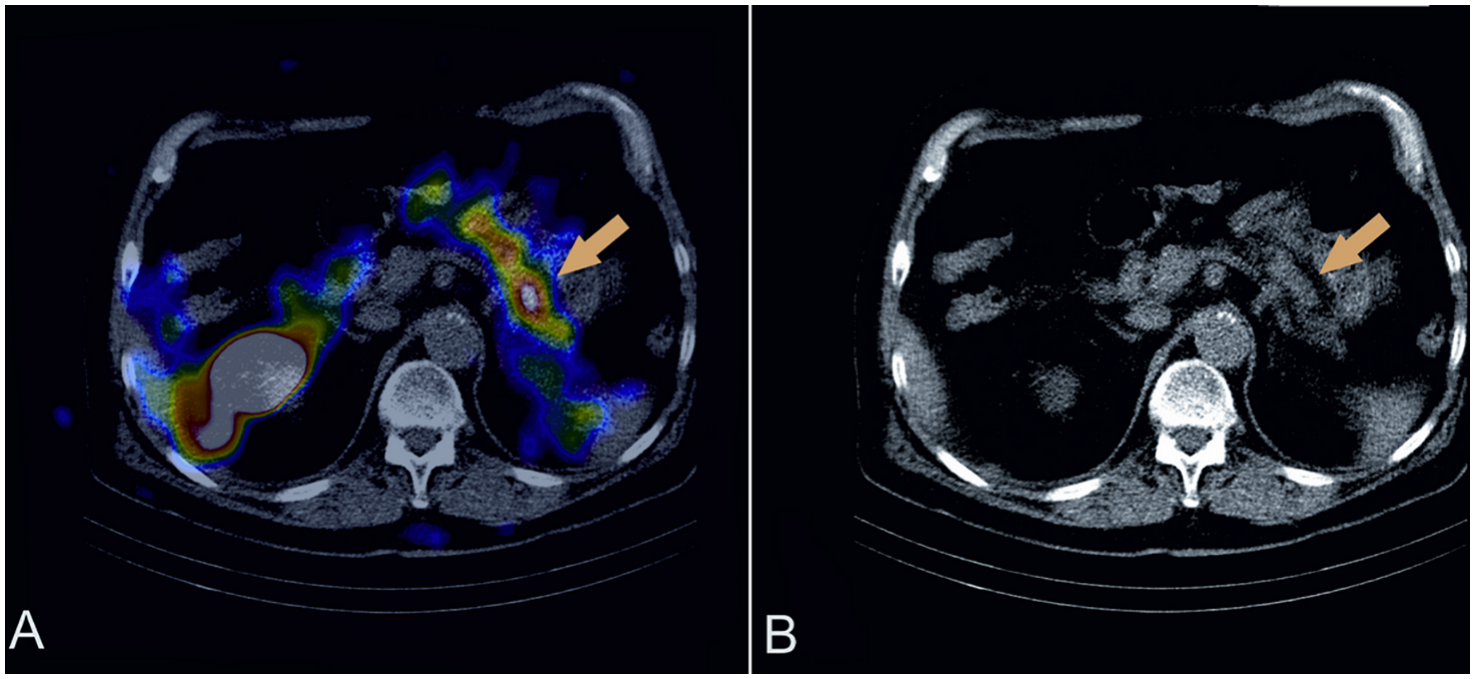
A 75-years old male with suspicion of insulinoma—^99m^Tc-GLP1scintigraphy. ^99m^Tc-GLP1scintigraphy revealed the focus of insulinoma in the pancreas—patient was disqualified from surgical excision of the tumor due to severe heart failure. The study was performed with dual-head, large field of view E.CAM gamma camera with low-energy high resolution (LEHR) collimators and images were evaluated with CT examination imposed by software fusion. A. Fusion of GLP-1 receptor imaging and CT—axial slice, B. CT—axial slice.
